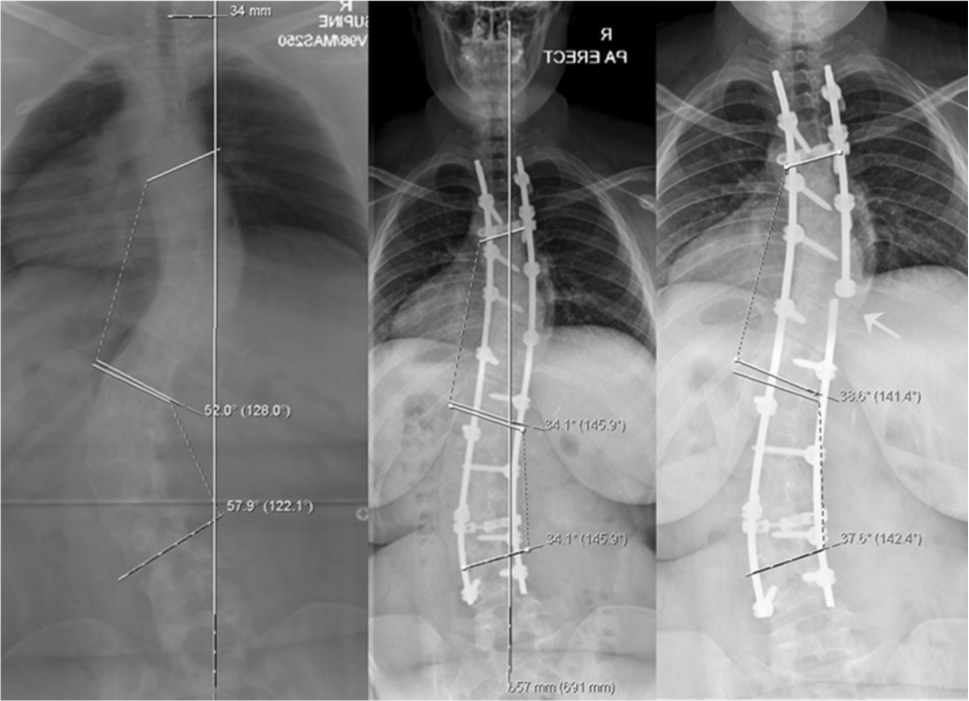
Adverse event – Radiographs of AIS with right rod breakage at 25 months following T3 – L4 PSFLate deep MRSA infection warranting removal of all instrumentation at 47 months post-op in a 15 year old male. Sound arthrodesis was seen at the time of implant removal and deep cultures grew MRSA (Fig 6).
